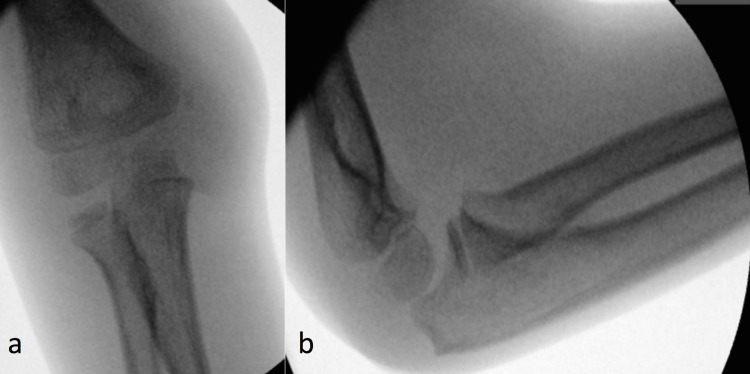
Intraoperative (a) anteroposterior and (b) lateral radiographs demonstrating satisfactory reduction of the posterior elbow dislocation, with no other osseous injury evident at this time. Undisplaced medial ossification centre noted.
